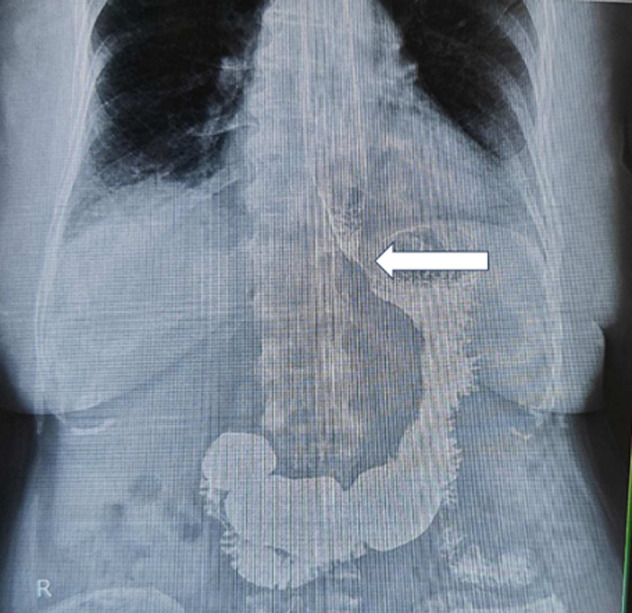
barium meal X-ray of esophageal atresia
